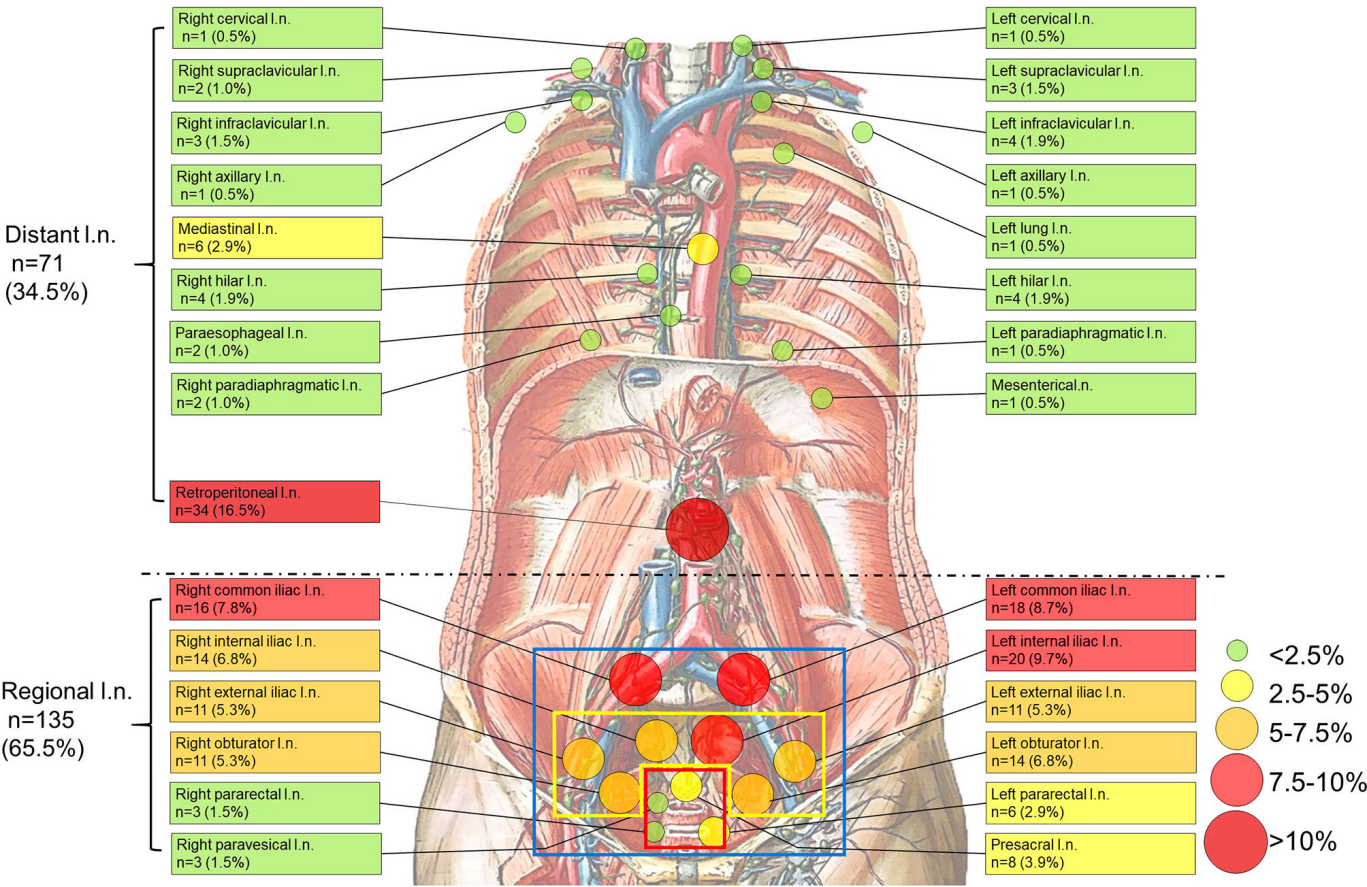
Anatomical distribution of PSMA-avid lymph node metastases in the whole body. (blue square, the scope of ePLND; yellow square, the scope of limited PLND; red square, the selective resected lymph nodes if radioactive in sentinel PLND). Anatomical distribution of non-regional PSMA-avid lymph node metastases. All non-regional lymph node metastases (n=71, 34.5%) are beyond pelvic mpMRI, the currently recommended imaging by the guidelines (7, 9). Background picture (Lymph Vessels and Nodes of Posterior Abdominal Wall) was used under the permission of ^©^ Elsevier Inc.
